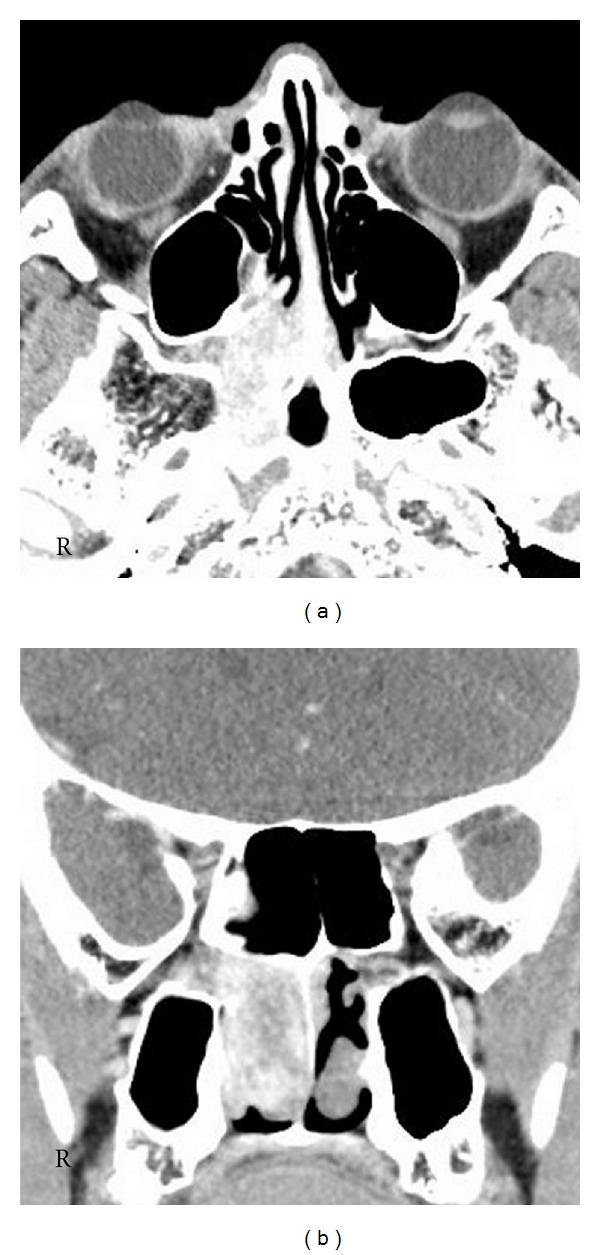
Enhanced axial (a) and horizontal (b) computed tomography (CT) images. Homogenous and strongly enhanced mass filling the posterior part of the right nasal cavity with extension to the pterygopalatine fossa (PPF) and sphenoid sinus.
